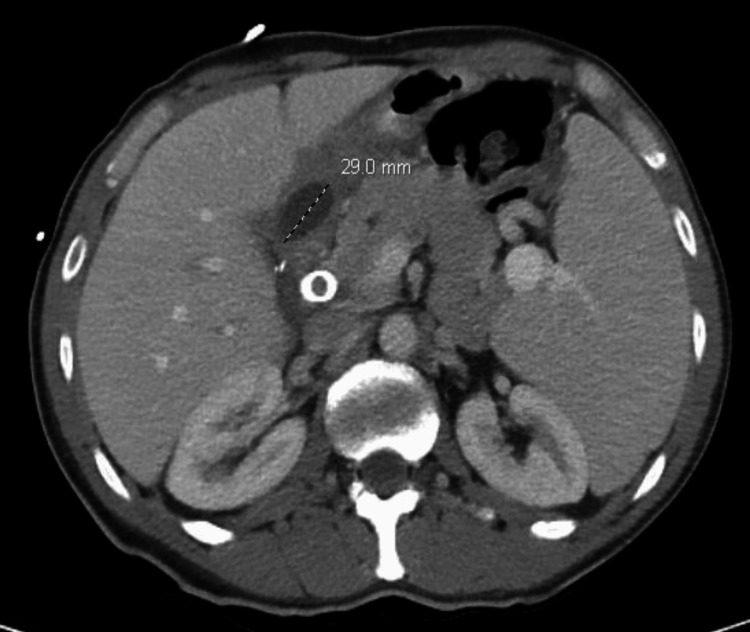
CT of the abdomen and pelvis with contrast (axial view): a 2.9 cm fluid collection near the right subhepatic region CT: computed tomography
